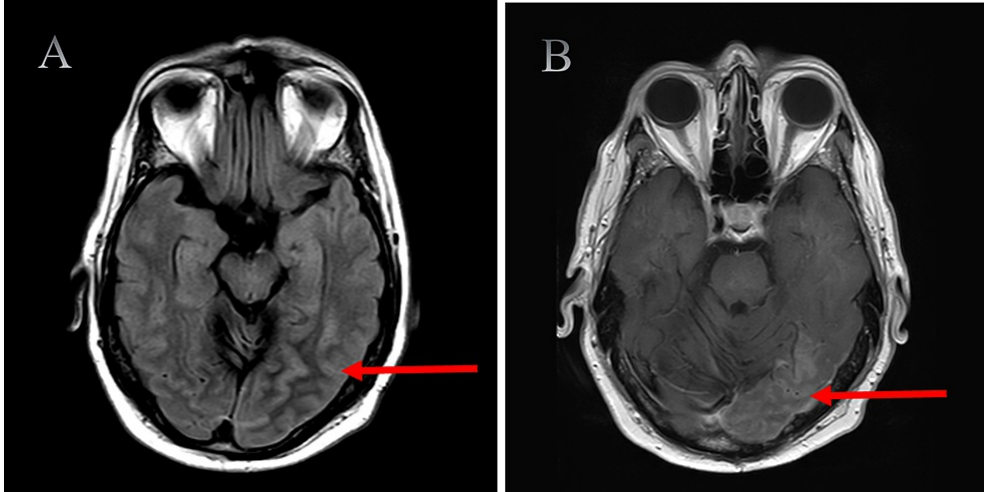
FLAIR axial T2 shows gyral edema in the left parietal and occipital regions (A) with associated gadolinium enhancement in the left occipital region (B) FLAIR: fluid-attenuated inversion recovery
